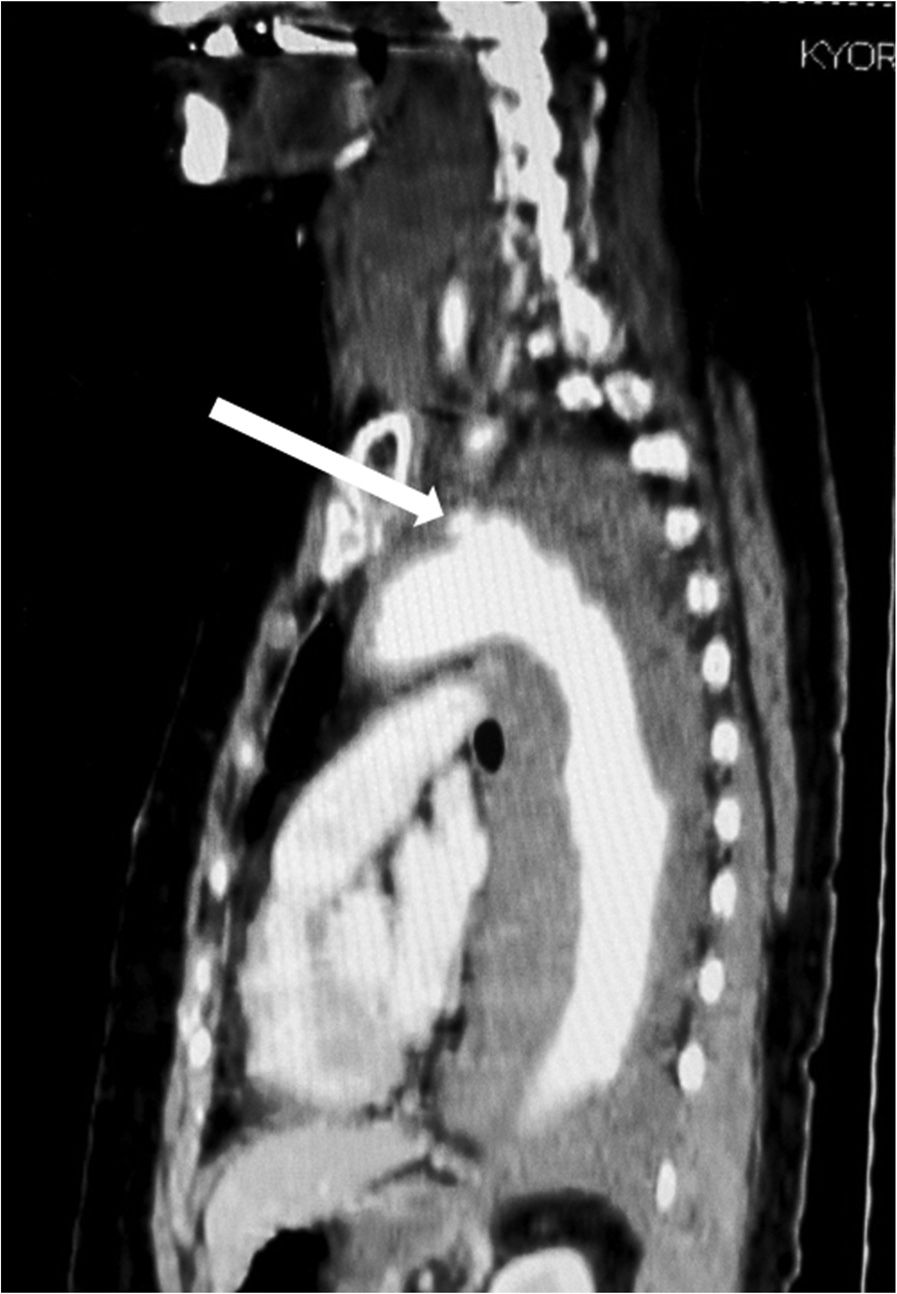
Reconstructed contrast-enhanced computed tomography scan. Dense atheromatous change was seen in the thoaracic aorta. A penetrating atherosclerotic ulcer was seen in the distal aortic arch (arrow).
